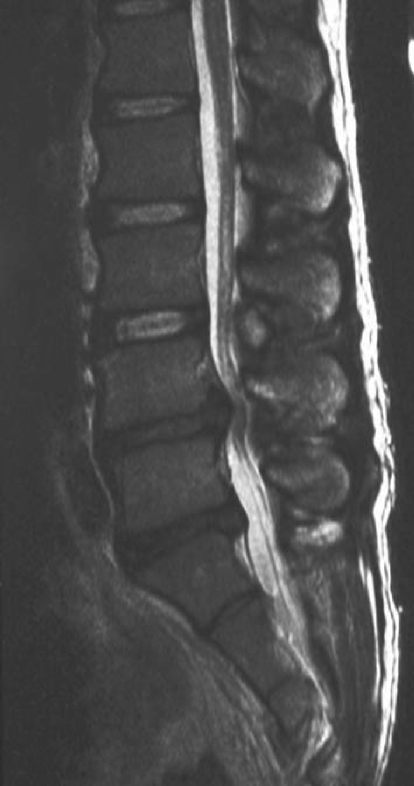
MR images of the lumbar spine revealed spinal canal stenosis at L4/5 (T2-weighted image, sagittal section).
